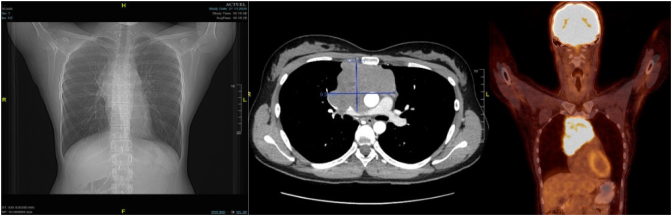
A: Thoracic X-ray antero-posterior view showing a slight enlargement of the upper mediastinum. B: Axial view of a pre-operative CT scan showing the lesion in the anterior mediastinum and an important mass effect. C: Thoracic PET scan in all three orientations showing the hypermetabolic anterior mediastinal lesion.
